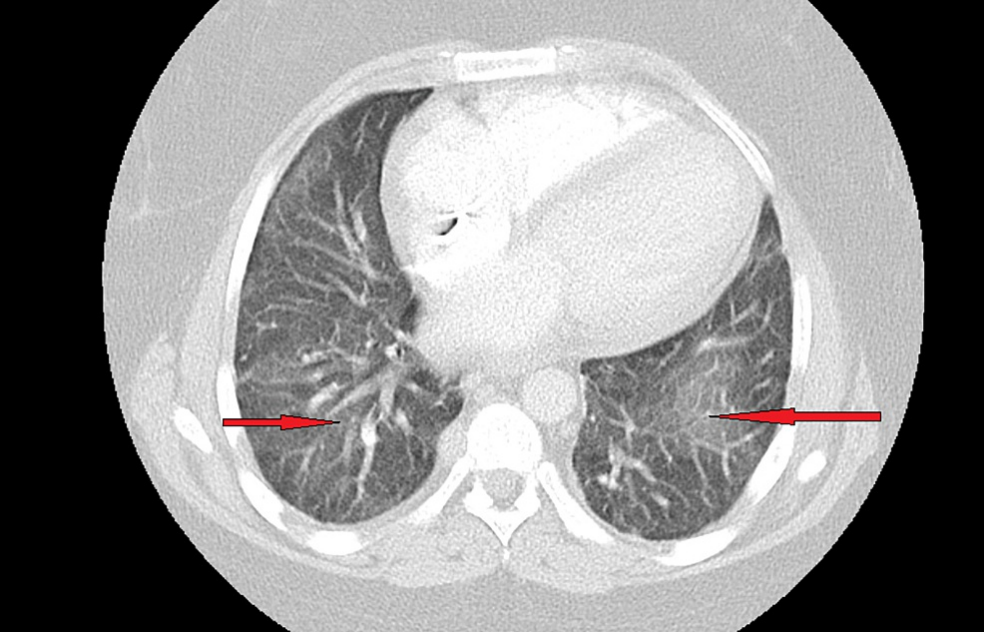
CT angiogram of chest showing patchy ground glass opacities at bilateral lung bases
